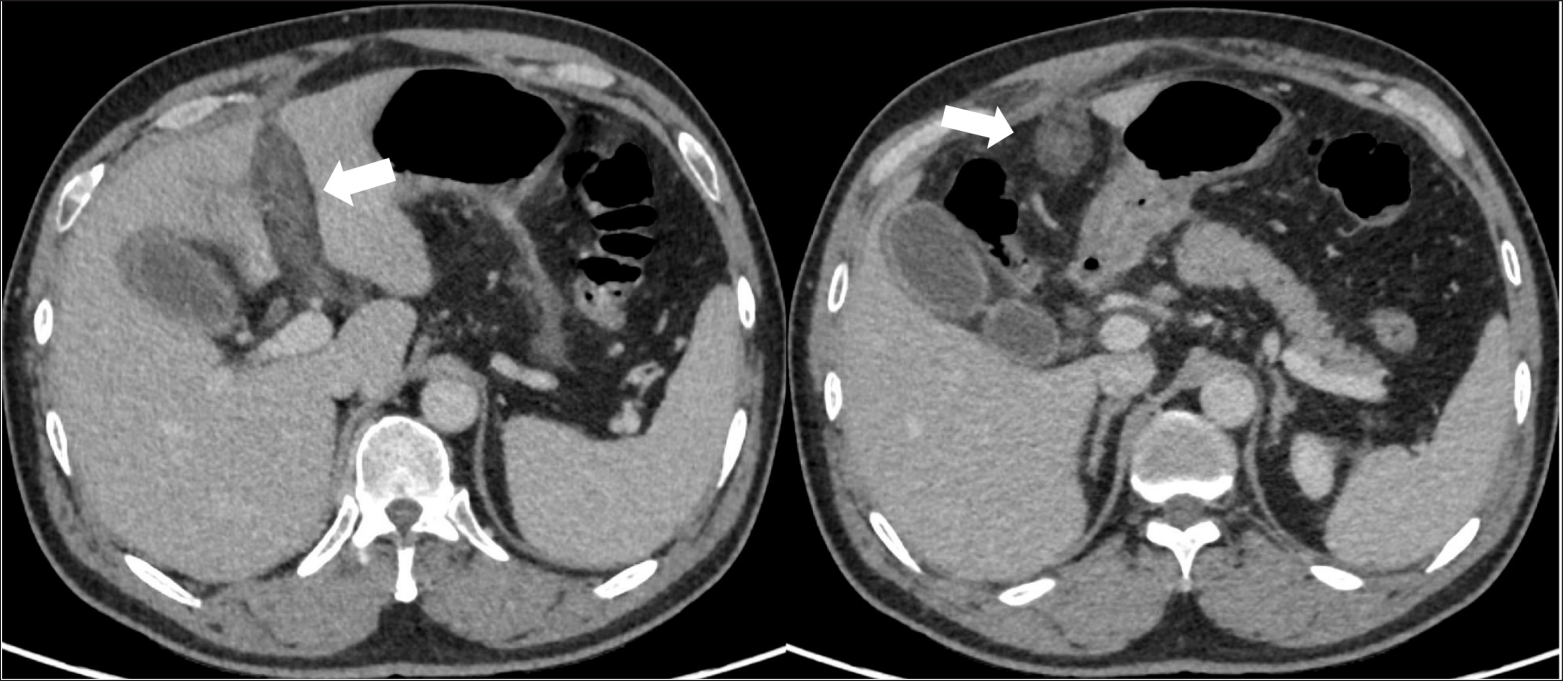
CT results suggested the diagnosis of necrosis of the ligamentum teres hepatis and provided a CT volume reproduction of the lesion to show its neighboring relationships (Figure 3, white arrow). To avoid serious complications, the clinician removed the lesion with minimally invasive surgery via laparoscopy and pathology results confirmed the diagnose of PNLTH.
